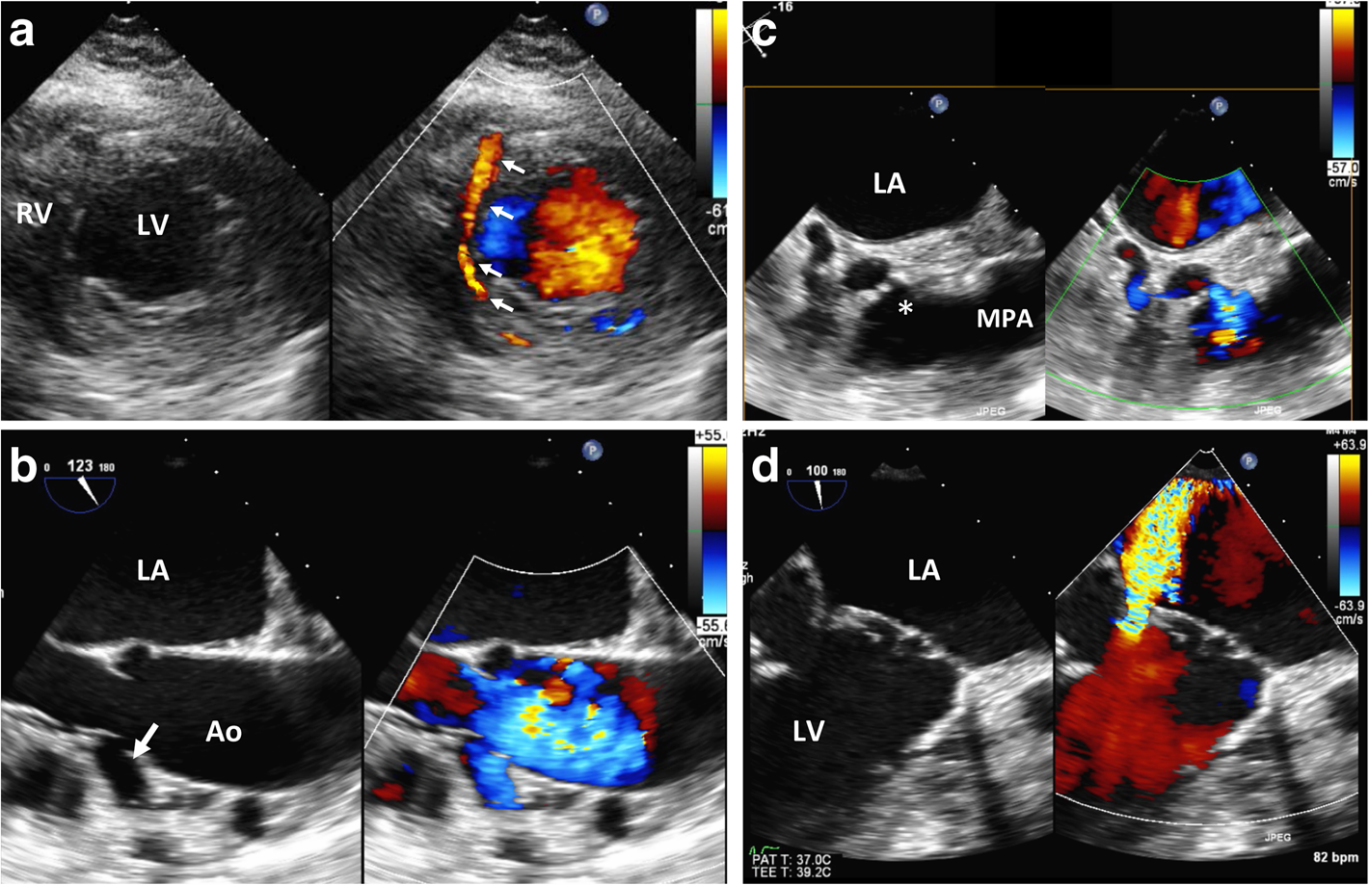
a Transthoracic echocardiography on short-axis view demonstrates an unusual, tubular-like structure inside the interventricular septum (arrows). b to d Transesophageal echocardiography reveals markedly dilated right coronary artery (RCA) arising from the right aortic sinus (arrow, b). There was a tortuous, abnormal vessel located adjacent to main pulmonary artery (MPA) emptying into the posteromedial aspect of the MPA with accelerated flow across the stenotic ostium (asterisk, c). Severe mitral regurgitation from posterior mitral leaflet restriction in combination with mitral annular dilatation is observed (d). LV; left ventricle, RV; right ventricle, LA; left atrium, Ao; ascending aorta
